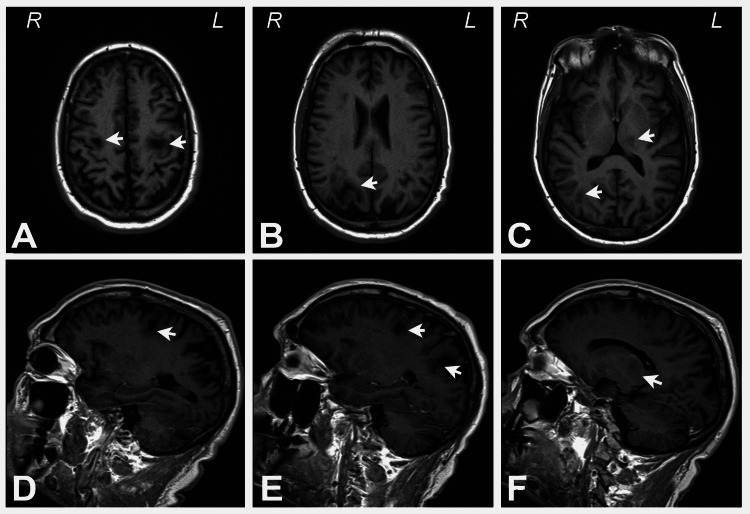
Unenhanced T1 FLAIR axial and sagittal images demonstrating the bifrontal, parietal, and bilateral thalamic lesions Top row: Unenhanced T1 FLAIR axial images demonstrating the (A) bifrontal, (B) right parietal, and (C) left thalamic and right thalamic lesions (arrows). Bottom row: Unenhanced T1 FLAIR sagittal images showing the (D) left posterior frontal, (E) right posterior frontal and right parietal, and (F) left thalamic lesions (arrows).
